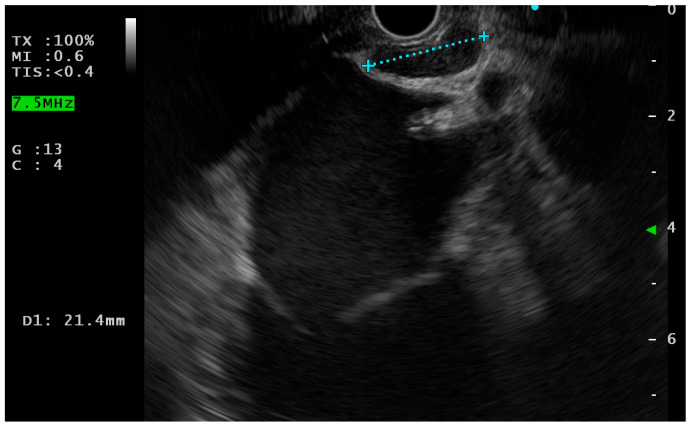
Endoscopic ultrasound (EUS) view of subcarinal space with a lymph node (21.4 mm of diameter).
